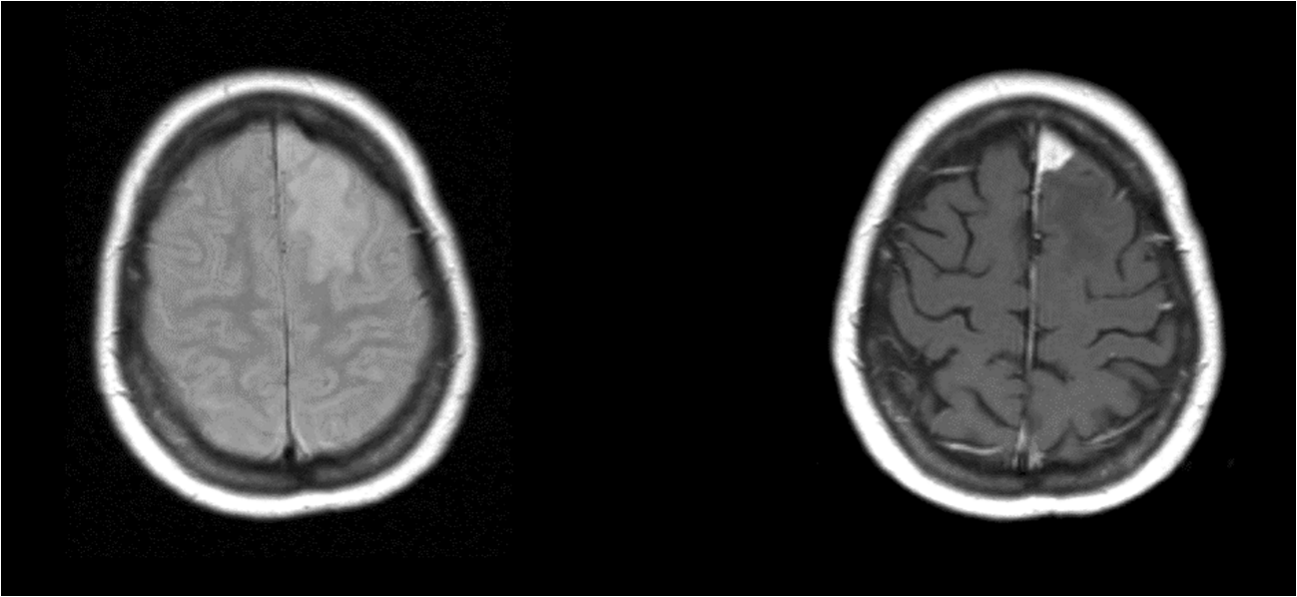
Magnetic resonance images. Meningioma with edema. Left image T2-weighted image; right image T1-weighted image with gadolinium
